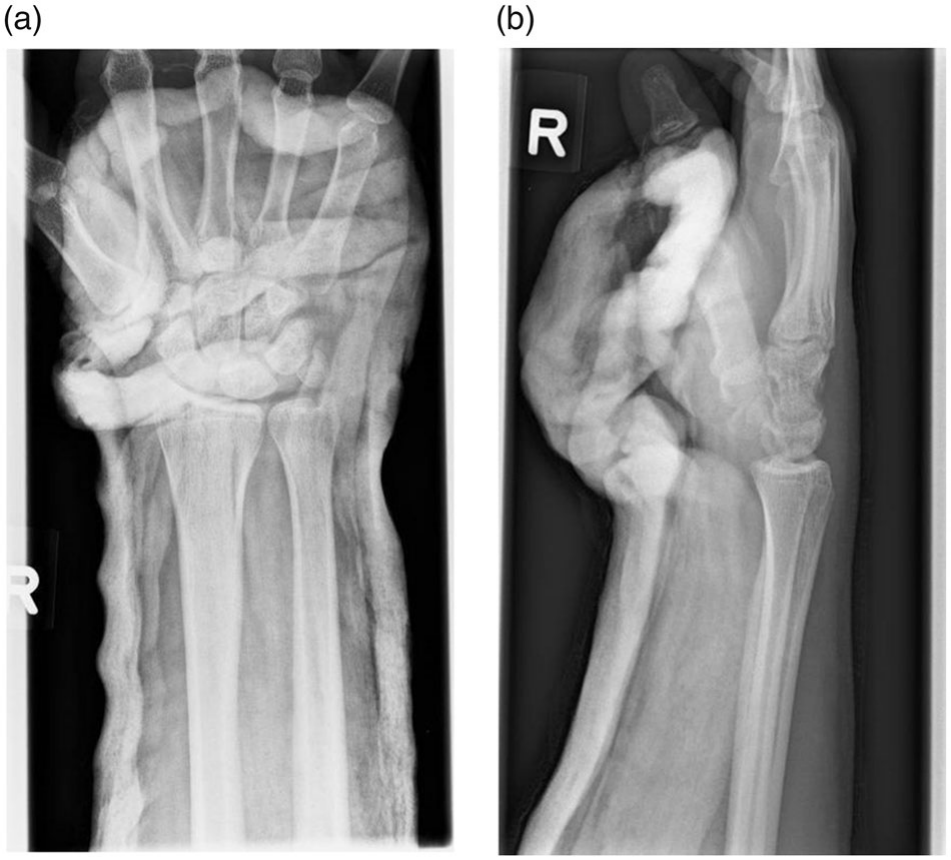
(a) X-ray (anteroposterior view) of the right hand after an unsuccessful manual closed reduction. (b) X-ray (lateral view) of the right hand after an unsuccessful manual closed reduction.
